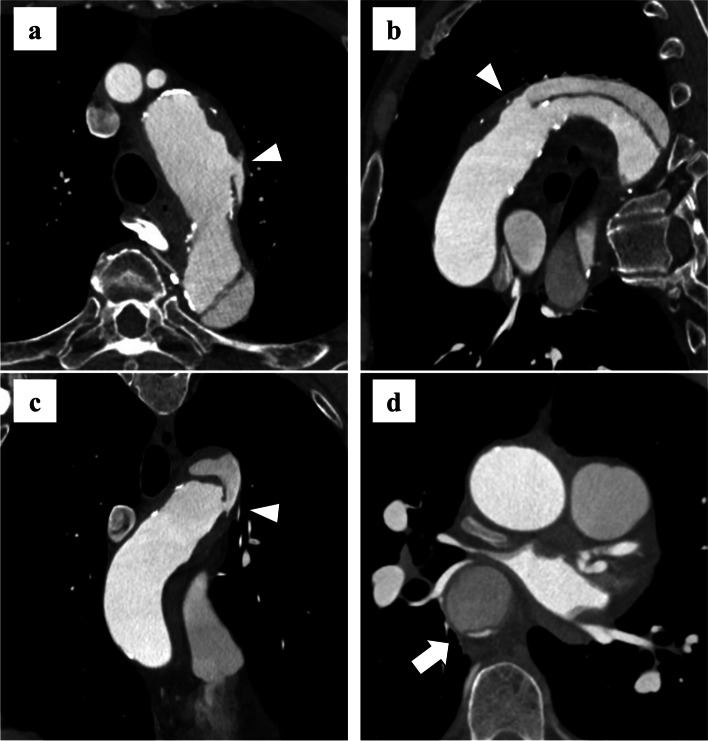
Contrast-enhanced computed tomography before thoracic endovascular aortic repair. Axial and multi-planar reconstruction (MPR) imaging reveals an acute type B aortic dissection. a–c The arrowheads indicate the primary entry located at the distal aortic arch. d The white arrow indicates the true lumen of descending and thoracoabdominal aorta severely compressed by the false lumen
